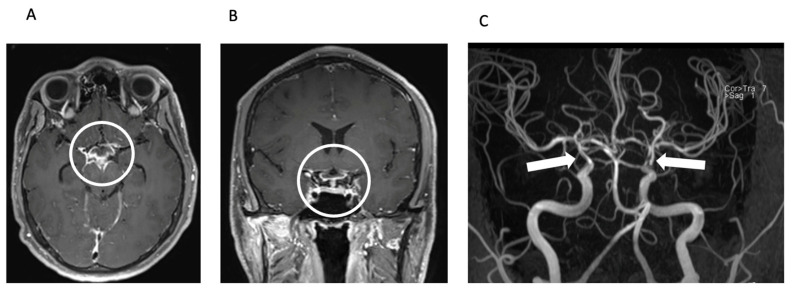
Meningitis and secondary CNS vasculitis on MRI. Axial (A) and coronal (B) reconstructions of contrast-enhanced T1 SPACE image showing basal contrast enhancement predominantly in the basal cisterns around the circulus of Willis (circle). TOF MR angiography (MIP, posteroanterior view—(C)) shows severe stenoses of the intradural proximal cerebral arteries, predominantly in the distal ICA including carotid T bilaterally (arrows).
